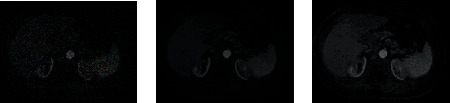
Comparison of MRI imaging with different methods. (a) Original image, (b) conventional MRI results, and (c) MRI results based on Retinex algorithm.
